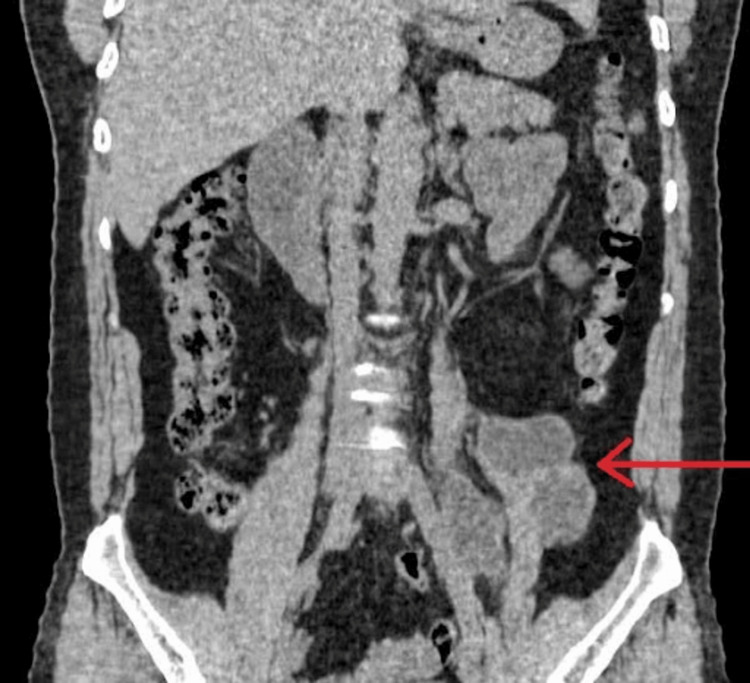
CT scan without contrast. Coronal view (red arrow) showing multiseptated left psoas cyst.
